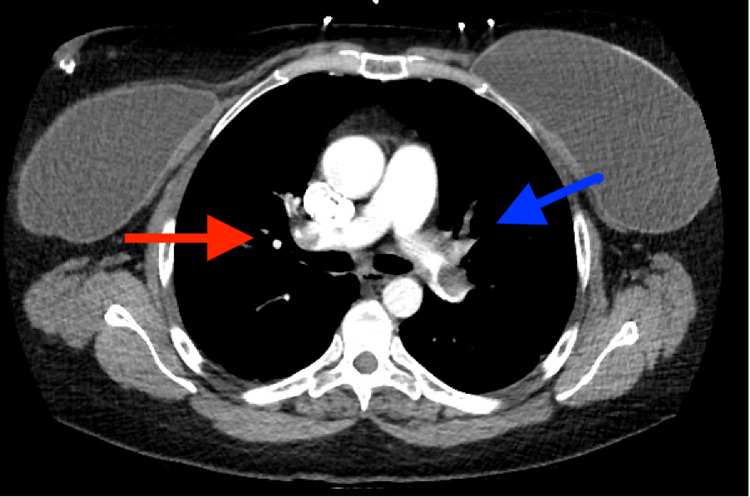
Pulmonary CTA demonstrating PE on the right (red arrow) and on the left (blue arrow). CTA, computed tomography angiogram; PE, pulmonary emboli
